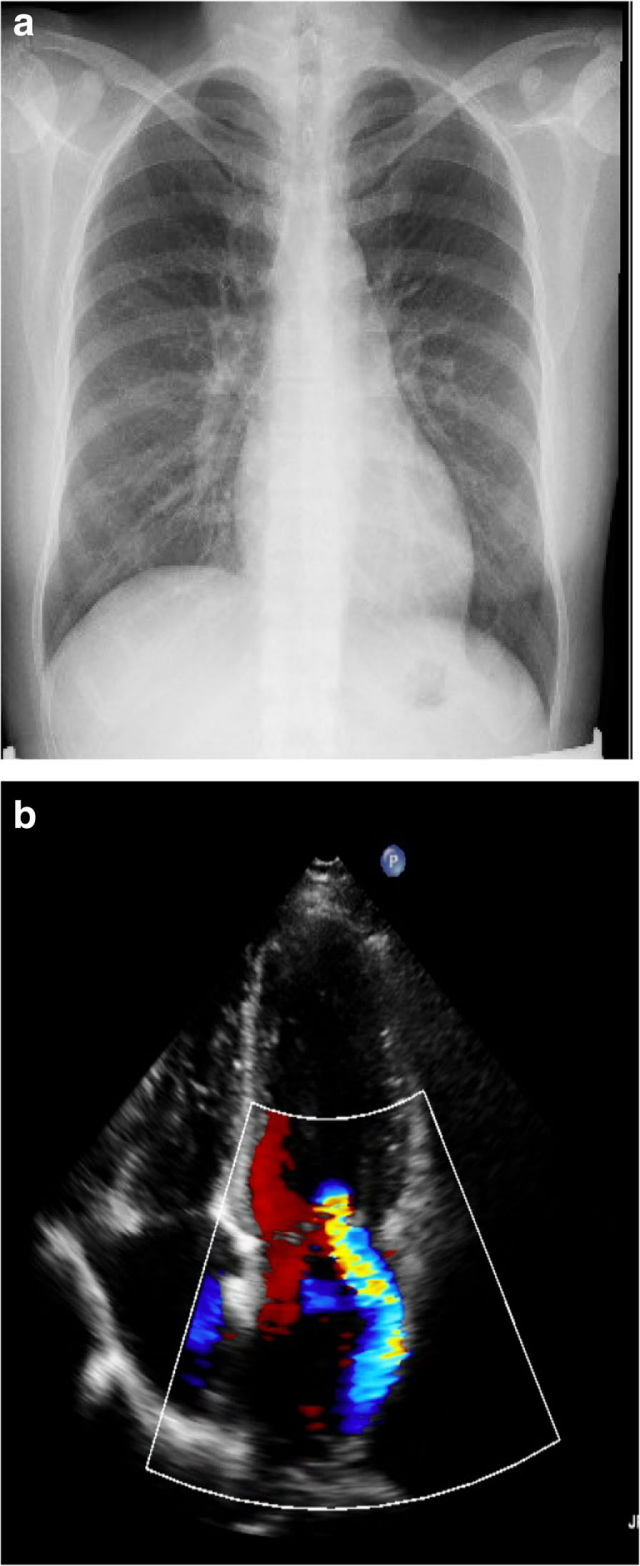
Preoperative examinations. a Chest radiograph showing no abnormality. b Four-chamber view of a transthoracic echocardiogram showing mild mitral regurgitation with tethering
